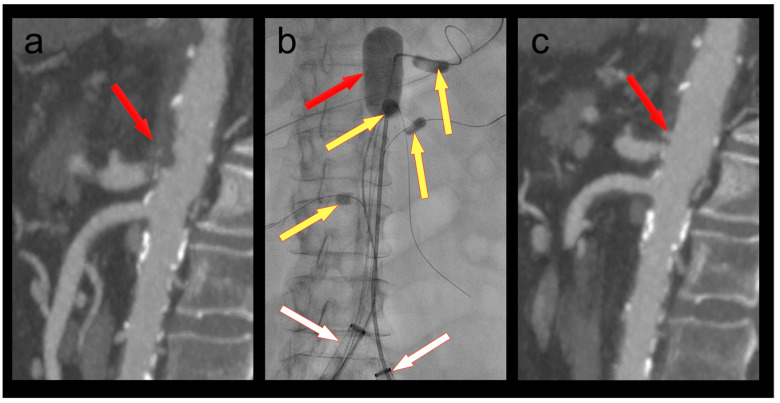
(a) Thrombus in the aorta and in the origin of the coeliac trunk (red arrow); (b) thrombectomy (red arrow) of the thoracoabdominal aorta under balloon protection of the coeliac trunk, superior mesenteric artery, and both renal arteries (yellow arrows). The large-bore sheaths are occlusive in both common iliac arteries (white arrows); (c) Postoperative CT scan with no residual thrombus in the aorta or the coeliac trunk (red arrow).
